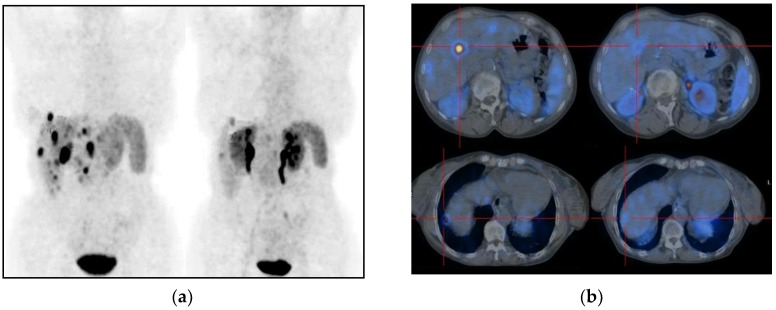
Example of [^68^Ga]-DOTA-NOC PET/CT before and after treatment with somatostatin analogs in a patient with metastatic ileal neuroendocrine tumors (NET). (a) Multi intensity projection (MIP) views of several liver metastases at staging (left) and after treatment (right); (b) transaxial images of liver showing the presence of metastasis (left) and their almost complete disappearance after treatment.
